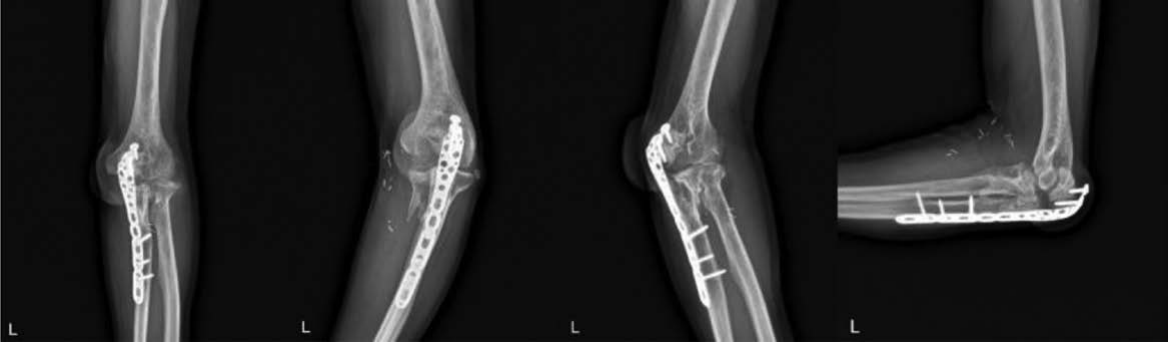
After 2 yr follow up, nonunion of olecranon can be seen in X-ray.
